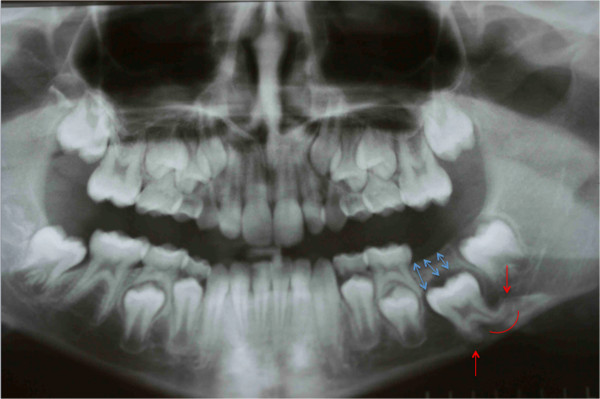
Second orthopantomograph prescribed to the patient by us before our intervention to assess the evolution of the clinical case and formulate a new plan of treatment: the situation was even worse; the roots were all sizes and showed closed apexes (red arrows), and the presence of bone above was increased with respect to the first evaluation (blue arrows).
